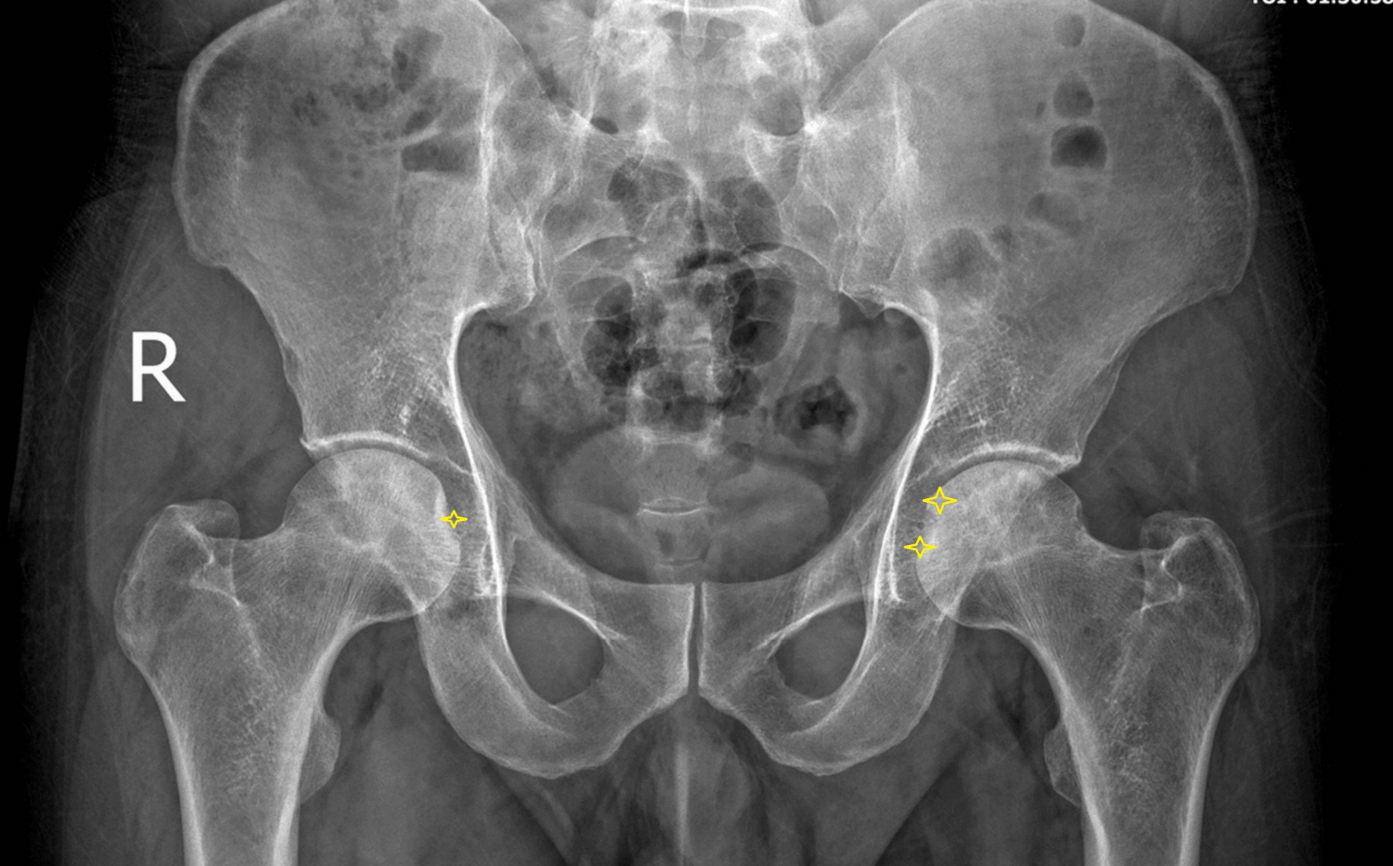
Anteroposterior (AP) pelvic radiograph of a 43-year-old male with sickle cell trait. A follow-up at three months revealed a faint, curvilinear radiolucent line parallel to the articular surface of the left femoral head, known as the "crescent sign" (asterisk). This finding indicates subchondral fracture and impending articular collapse, consistent with Ficat and Arlet stage III.
